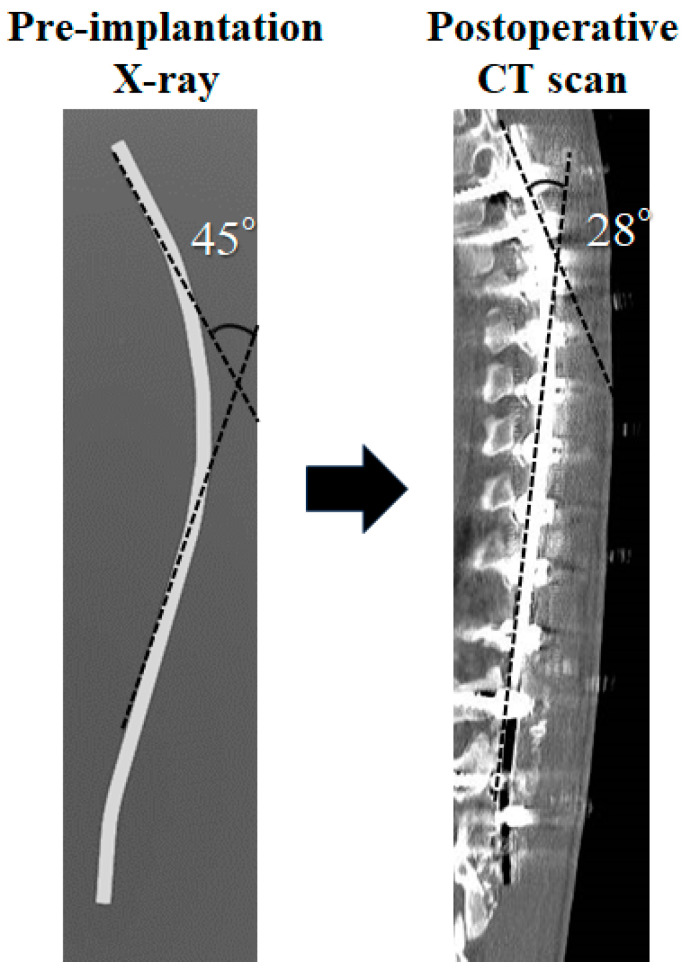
This figure shows concave rod deformation. The concave rod was 45° before surgery. CT scan showed deformations of 28° after surgery. The convex rod was not deformed.
